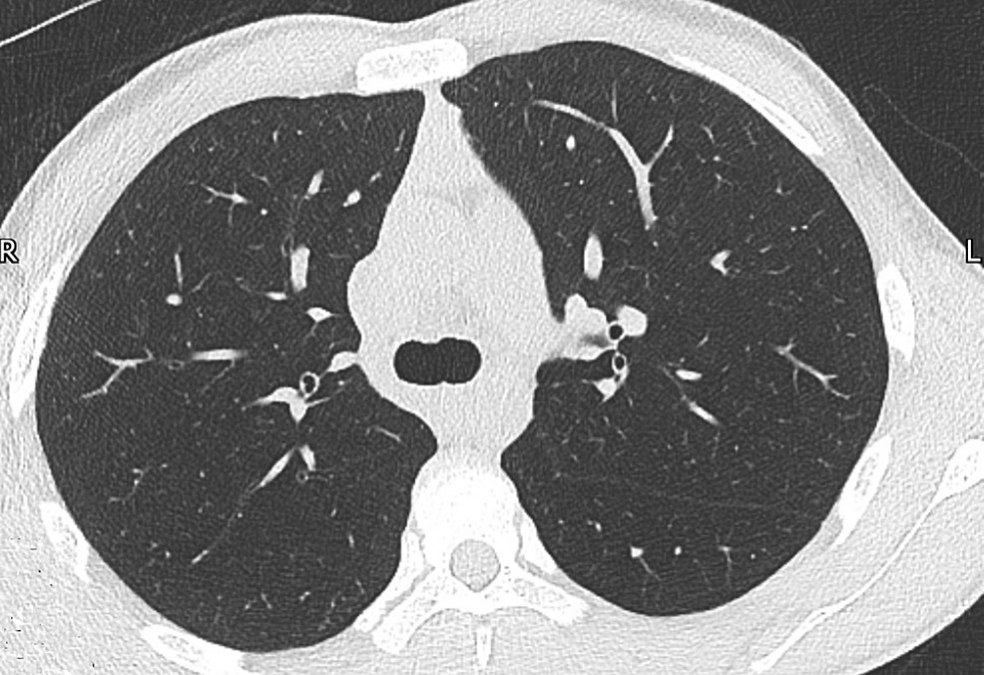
CT of the thorax image after four weeks showing resolution of pneumomediastinum.
